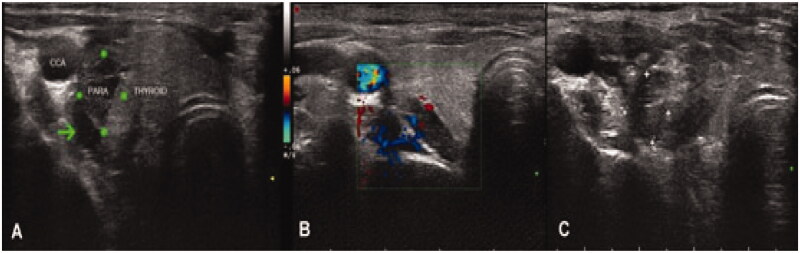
Images from a 29-year-old female diagnosed with severe SHPT. (A) Before MWA, a liquid insulation layer (the arrow head) was created to protect the adjacent recurrent laryngeal nerves and vessels from thermal injury. The size of the parathyroid gland at the superior right position detected by ultrasound was 1.7*1.3*0.6 cm. (B) Color Doppler image showed abundant blood flows in the gland before MWA. (C) After MWA，the local echo of the nodule was not uniform when detected by ultrasound. SHPT : secondary hyperparathyroidism.
